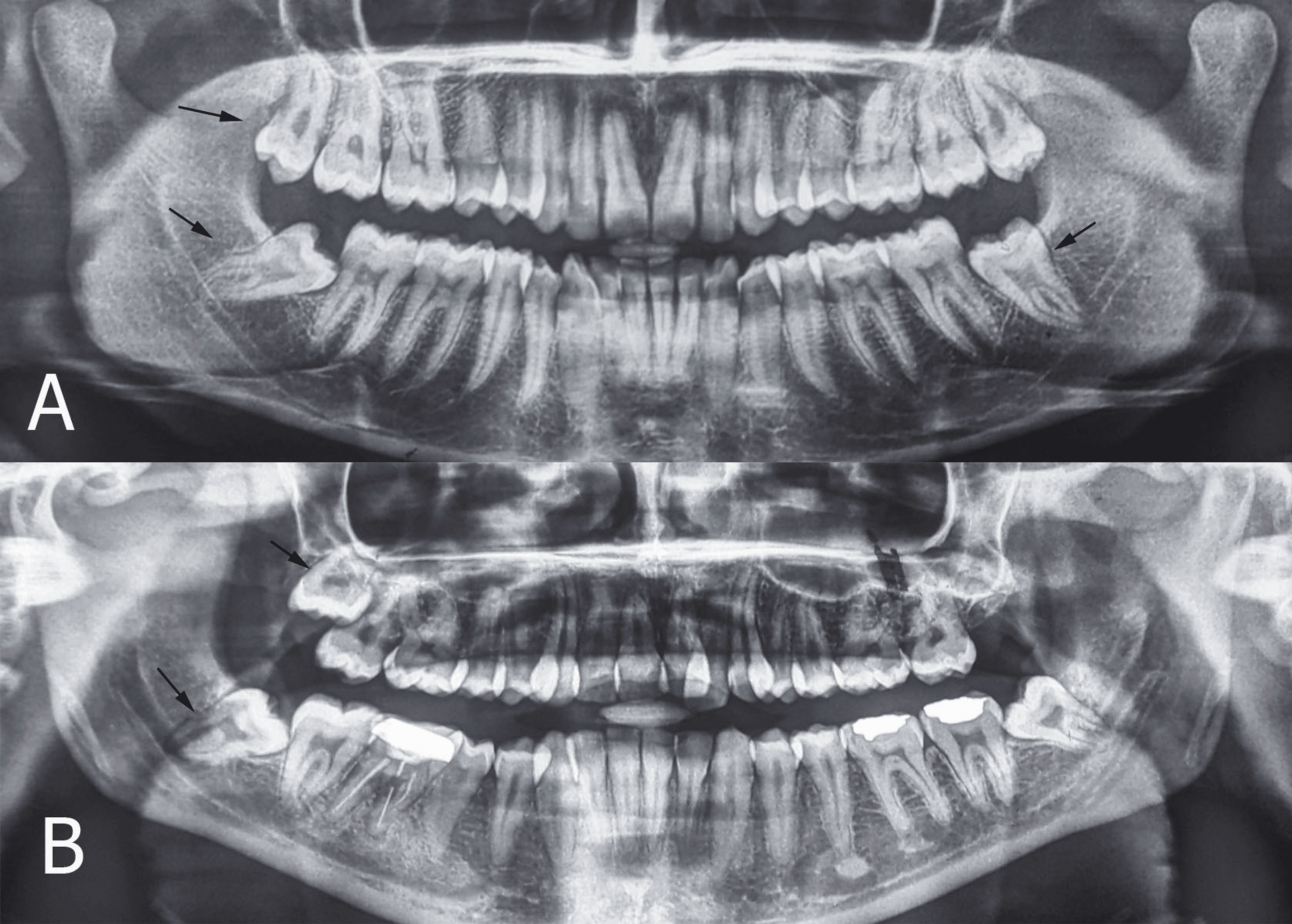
Orthopantomogram showing (A) impacted maxillary and mandibular third molar on the right side (difficulty index of 3 and 5, respectively) and (B) impacted maxillary and mandibular third molar on the right side (difficulty index of 5 and 6, respectively). Images are from patients included in the study, and the difficulty index was noted.
